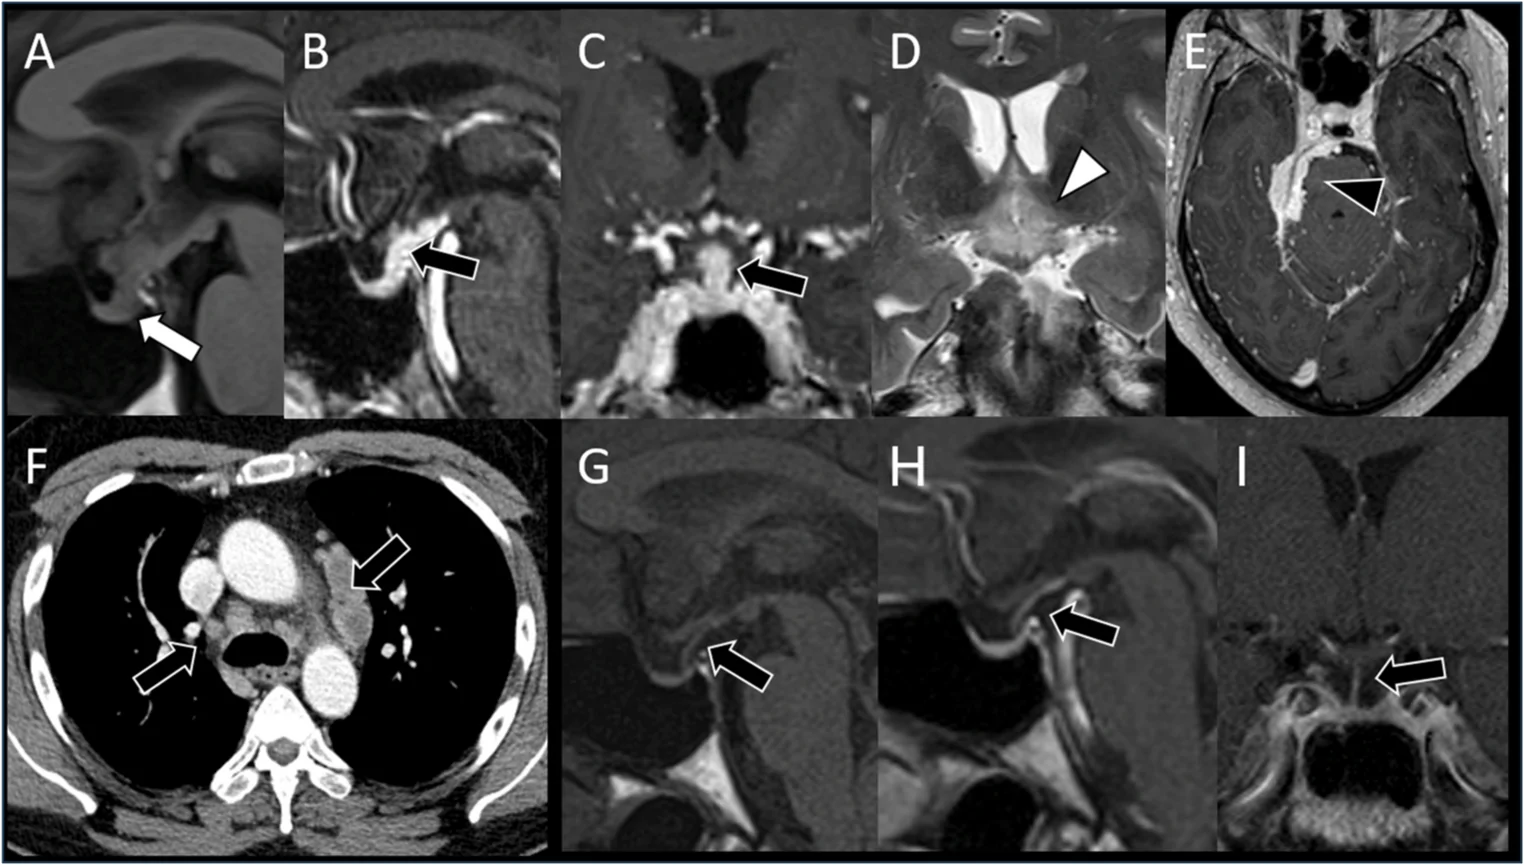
Sarcoidosis. A 45-year-old woman with headache and panhypopituitarism. Imaging shows a normal sella and absence of the posterior pituitary T1WI bright spot (A, white arrow). Contrast-enhanced images reveal a thickened stalk (B–C, black arrow) and pachyleptomeningeal enhancement (E, black arrowhead). Coronal T2WI demonstrates hypothalamus/optic chiasm edema (D, white arrowhead). Contrast-enhanced chest CT shows mediastinal lymphadenopathy (arrow). Two-year follow-up post-corticosteroid therapy shows normalization of the pituitary stalk (G–I, arrows)
